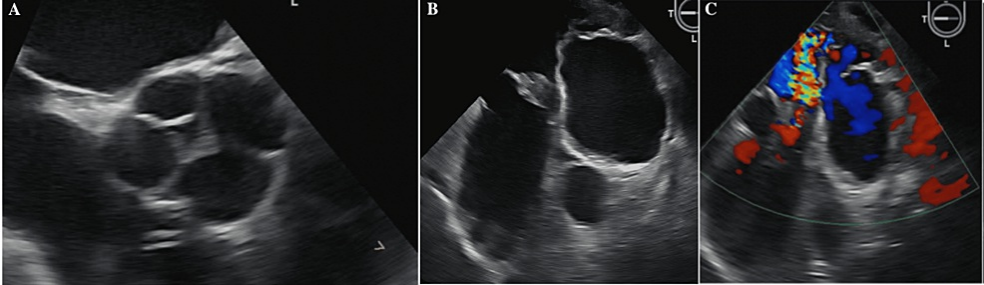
The transesophageal echocardiogram (TEE) revealed a unique abnormality of the aortic valve. The mid-esophageal aortic valve short-axis view (A) demonstrates a quadricuspid aortic valve (QAV). These leaflets also exhibit thickening at their tips, and a concerning 1.3 x 1 cm mass is attached to one of them (visible in both A and potentially B). Furthermore, the long axis view of the left ventricle (C) shows mild to moderate aortic regurgitation.
